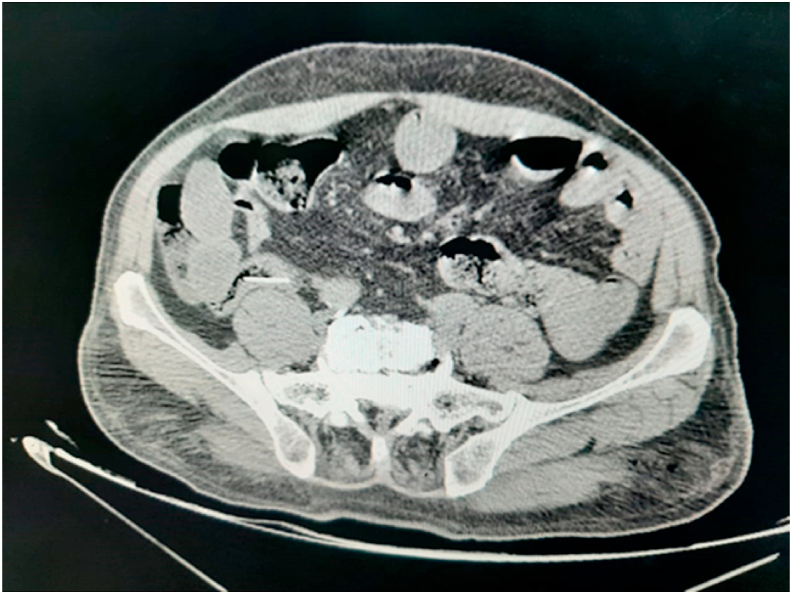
Axial contrast-enhanced CT-scan of the abdomen showing a fish bone.
